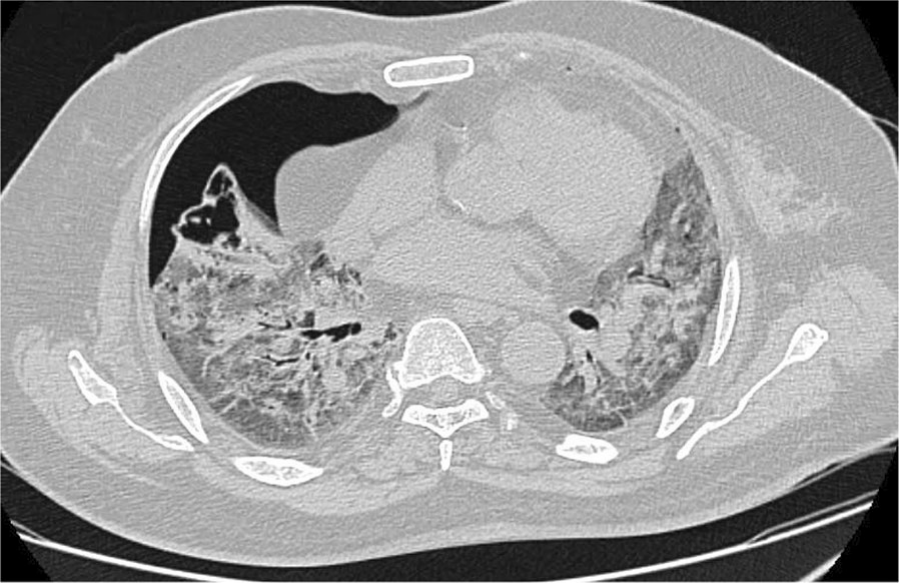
Axial HRCT scan of a 52-year-old male revealed pneumothorax on the right side. A cavitary lesion with irregular margins and few internal septations was detected in the right middle lobe, indicating the likelihood of a fungal infection within the cavity. A culture test revealed that the patient had candidiasis. Mechanical ventilation was the cause of the pneumothorax. Cavity formation might be caused by invasive candidiasis, mechanical ventilation, or a combination of the two
